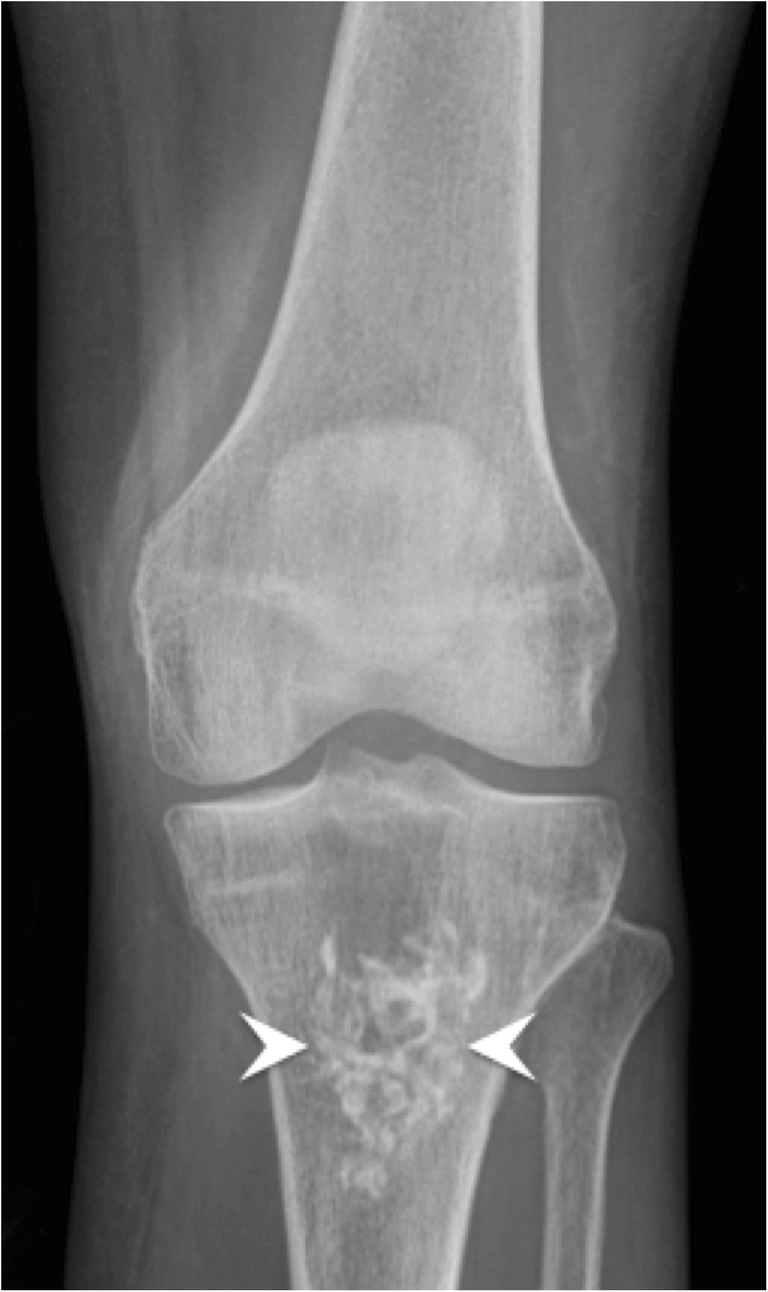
Enchondroma. Anteroposterior radiograph of the left knee shows a typical chondroid matrix mineralisation with arcs and rings in the tibia (arrowheads)
